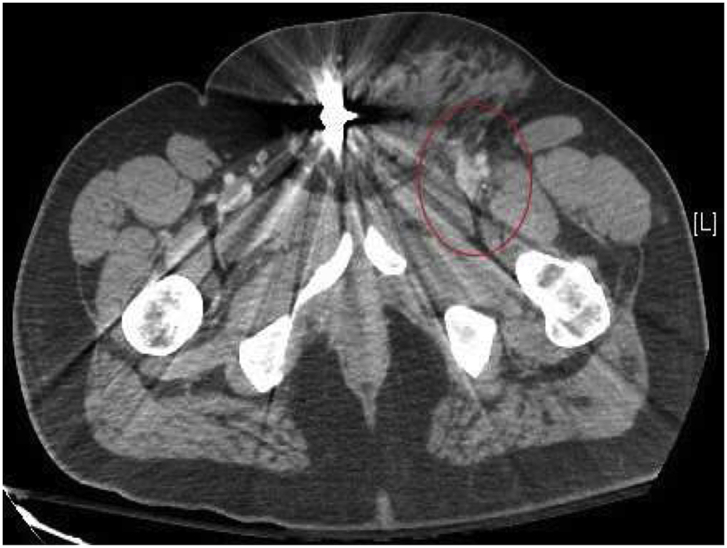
CT axial image of the left traumatic arteriovenous fistula between the deep femoral artery and common femoral vein with the associated perivascular tissue injury.
